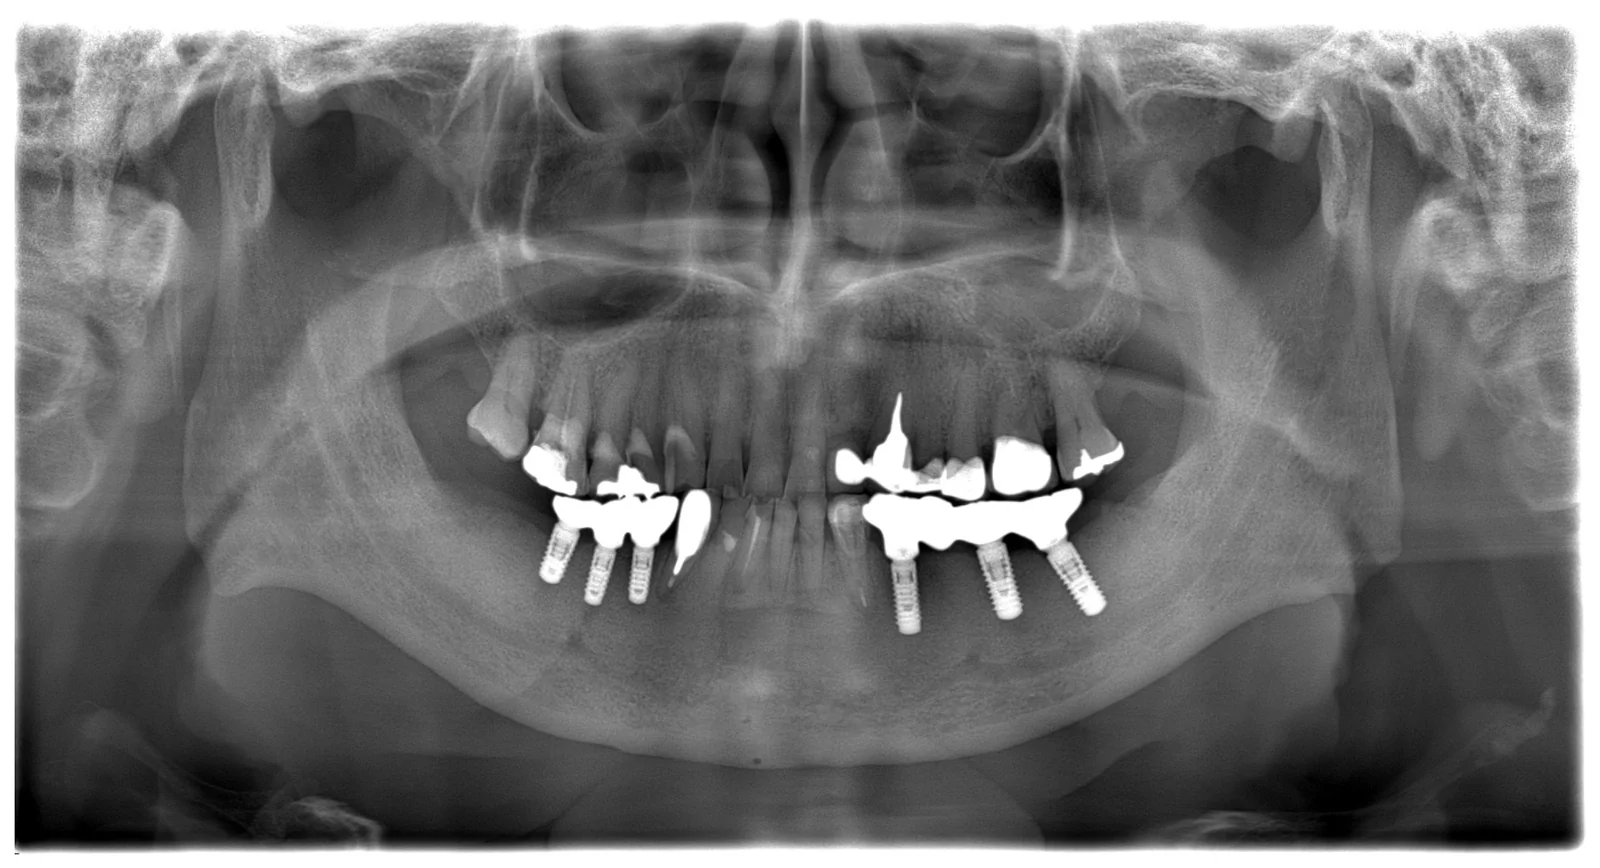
Panoramic radiograph obtained on the day of delivery of the definitive prosthesis (8 June 2026). The reimplanted contralateral #36 site is also visible, without abnormal findings.
